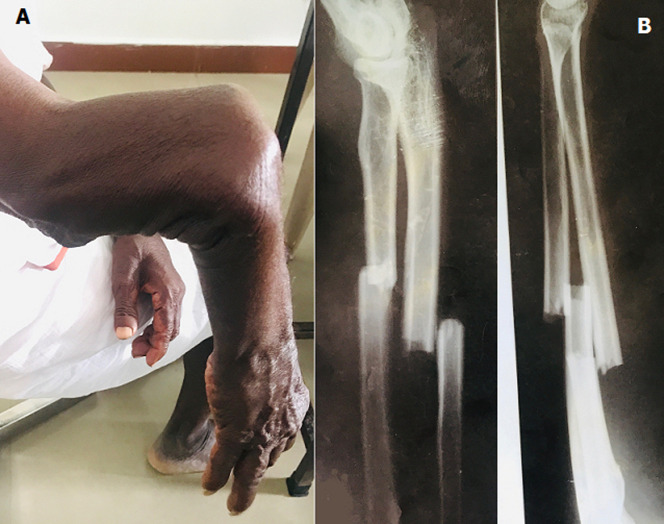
A) forearm with fracture nonunion; B) X-ray showing fracture both bones in forearm
